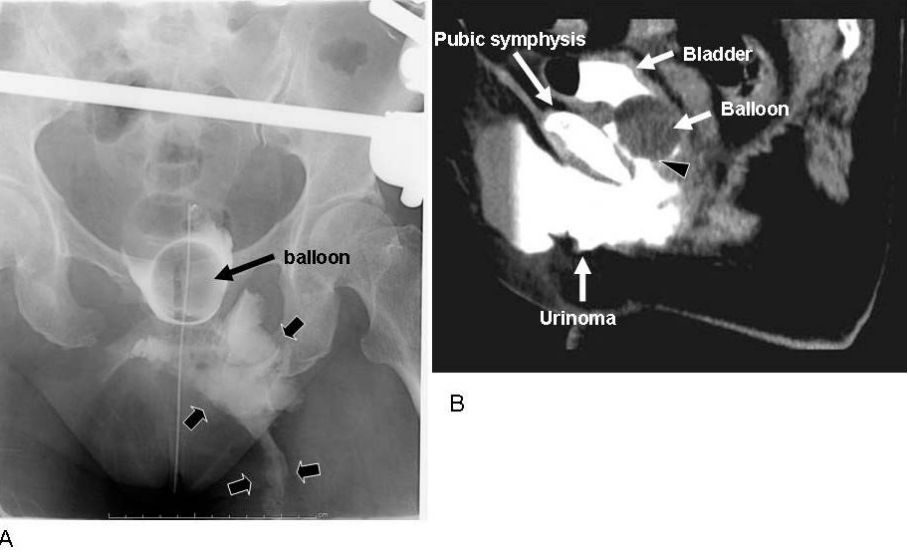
Retrograde cystography (A) performed on day 4 and sagittal reconstruction image of CT cystography (B) performed later. A: Leakage of extraperitoneal contrast medium outside the bladder is observed (arrows) B: In the sagittal reconstruction CT image, leakage of extraperitoneal contrast medium from the site shown by arrowheads is observed. The base of the bladder is positioned at the pubic bone inferior margin.
